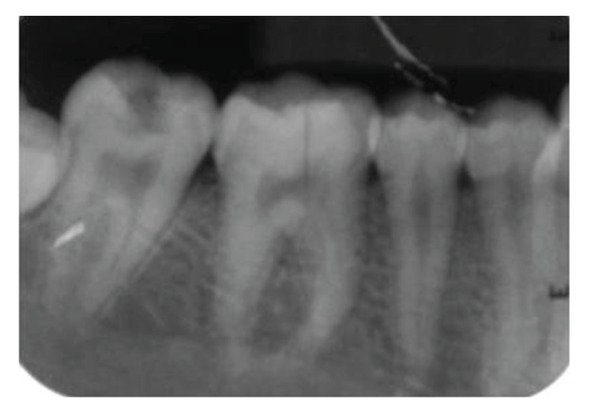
Preoperative periapical radiograph with 46 revealing radiolucent fracture line in the coronal aspect of the tooth.
